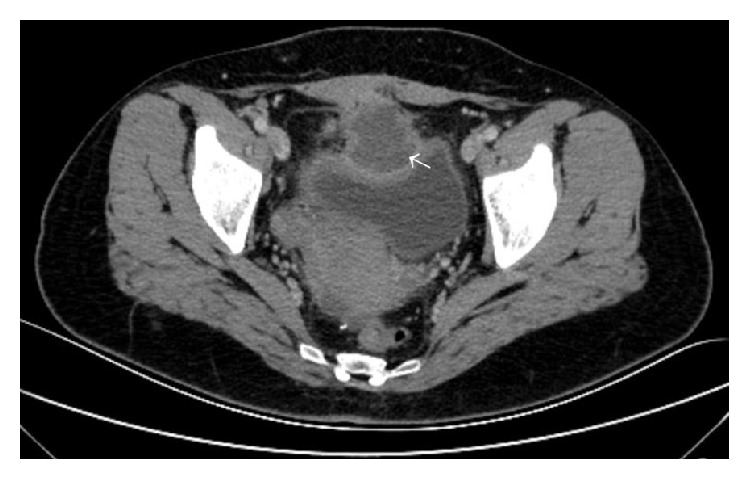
Abdominopelvic computed tomography showing urachal abscess (axial view).
